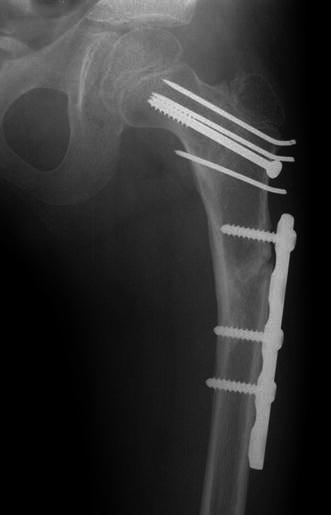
After removing the cast at two months postoperatively, the radiograph showed a 10° varus deformity of the subtrochanteric fracture with proximal screw back-out
